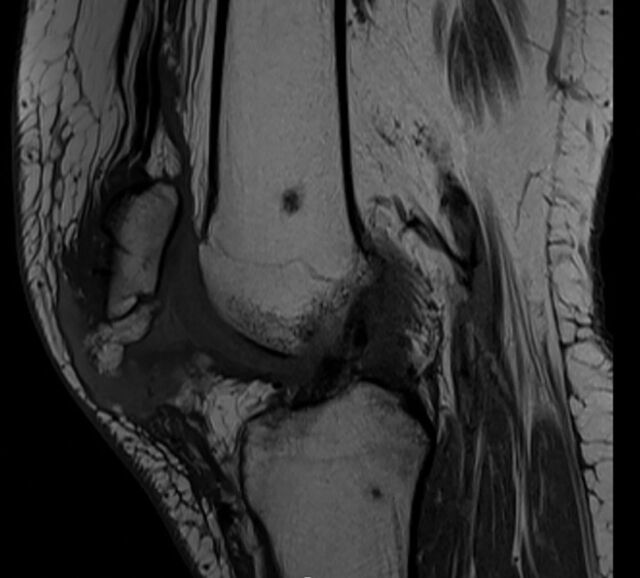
Sagittal T1 magnetic resonance imaging shows a full-thickness tear of the left patellar tendon with retraction.
